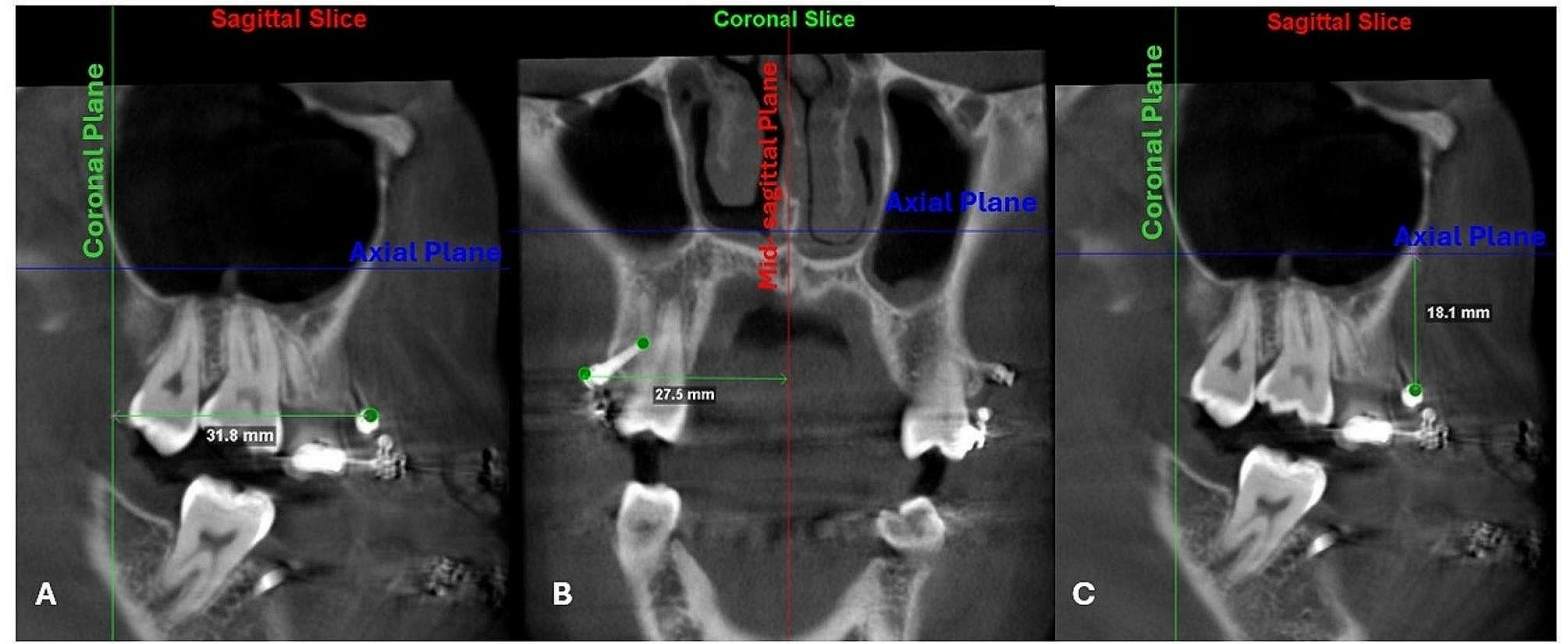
Cone beam computed tomography cross-sections showing the orientation of the three planes and measurement of the distances of minicrew’s head to planes: A, HMIC – coronal plane (green); B: HMIS – mid-sagittal plane (red); C, HMIA – axial plane (blue); (Dolphin Imagings software, version 11.95)
